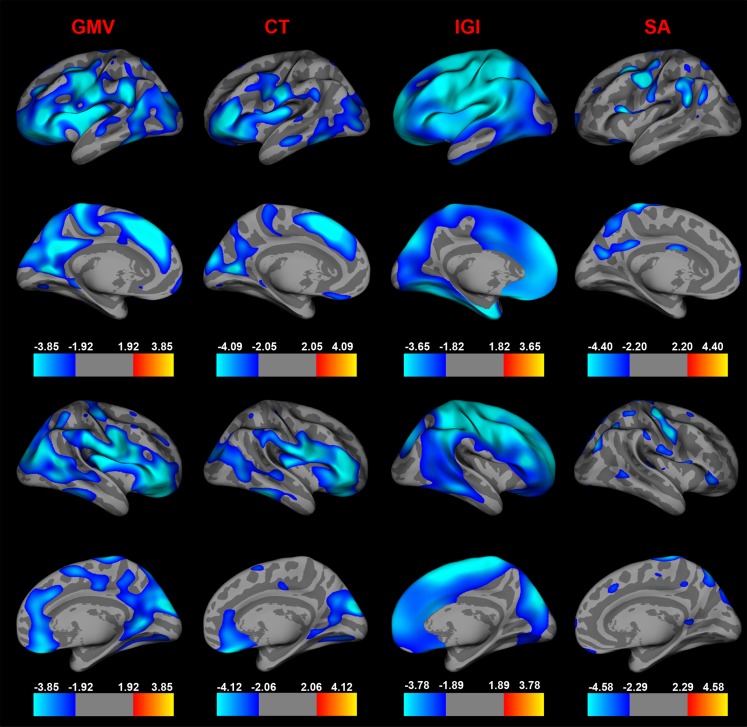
Comparison of Age-Related Changes between GM-Volume, Cortical Thickness, Cortical Surface Area and Gyrification. Age effects in a vertex-by-vertex analyses on GM-volume (GMV), cortical thickness (CT), lGI and cortical surface area (SA) presented on an average template brain. Left hemisphere from lateral view in the first row, from medial view in the second row. Right hemisphere is viewed from lateral view in the third row; medial view in the fourth row. The analyses for all parameters (lGI, cortical thickness, GM-volume and cortical surface area) were corrected for multiple comparisons with a false discovery rate (FDR) of q at 0.05 and to increase the signal to noise ratio, a 20 mm full-width at half maximum (FWHM) smoothing was employed. Blue colors indicate a significant decrease of lGI-, cortical thickness-, GM-volume- and cortical surface area- values with increasing age, whereas warmer colors are coded for age-related increases. Cortical thickness reductions showed the largest age-dependent effects in frontal, temporal and parietal regions (left hemisphere (effect sizes, Cohen's d): superior-frontal cortex: d = 2.27, rostral-middle frontal cortex: 1.84, pericalcarine: d = 1.29, middle-temporal gyrus: d = 1.01; right hemisphere: precentral cortex: d = 1.07, cuneus: d = 1.07, inferior temporal cortex: d = 1.15, superior frontal gyrus: d = 0.56) which overlapped with decreased GM-volume (left hemisphere (effect sizes, Cohen's d): superior-frontal: d = 0.78, superior-temporal: 1.46, lingual gyrus: d = 0.48, post-central gyrus: d = 0.81; right hemisphere: pars orbitalis: d = 0.59, inferior parietal: d = 1.39, paracentral gyrus: d = 0.82, superior frontal: d = 1.12). Cortical surface area decreased most prominently in parietal, precentral, supramarginal and caudal-middle frontal cortices (left hemisphere (effect sizes, Cohens'd): precentral cortex: d = 0.7, caudal-middle-frontal: d = 0.34, supramarginal: d = 0.45; right hemisphere: precentral cortex: d = 0.67, superior parietal: d = 0.69 and inferior parietal: d = 1.01).
